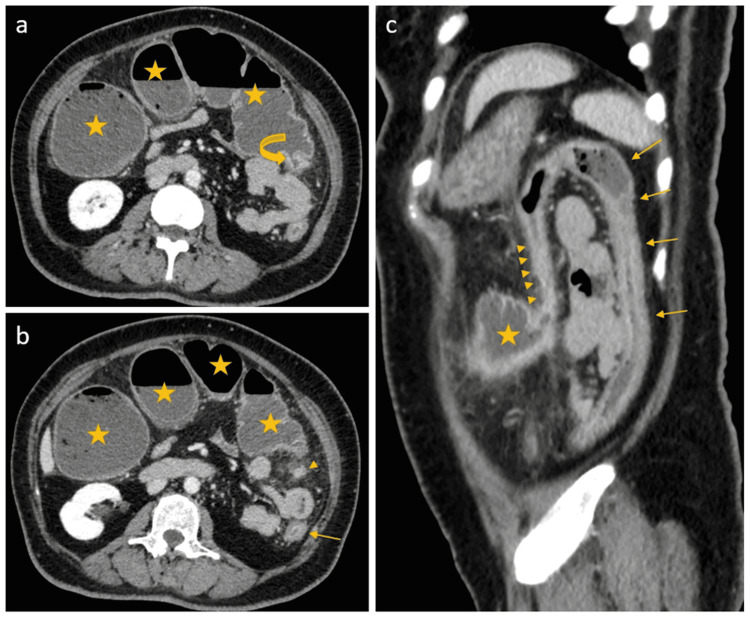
Interval contrast-enhanced abdominal CT scan. Axial (A & B) and sagittal (C) images show diffuse dilation of the ascending and transverse colon (star) with an abrupt cut off at the distal part of transverse colon showing a short segment of tightly narrowed hyperenhancing short segment of the splenic flexure (arrow heads) with surrounding fat stranding representing the transitional zone which is followed by collapsed distal colon (arrows) with faint surrounding fat stranding.
